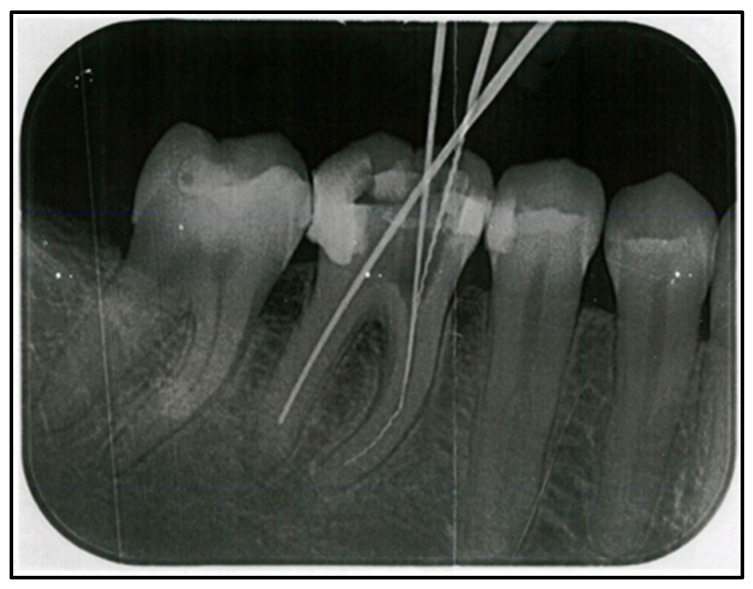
Pre-operative periapical radiograph of tooth 46 with three files taken at the dental practice during root canal treatment.
